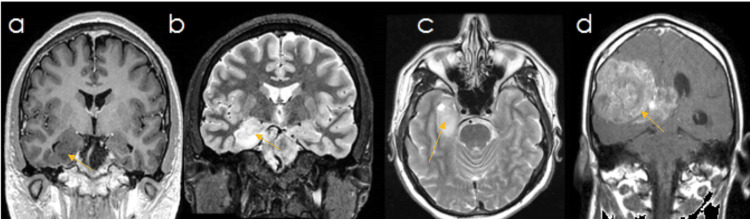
Radiological progression in patient 2 MRI: magnetic resonance imaging; FLAIR: fluid-attenuated inversion recovery Radiological progression in a two-month follow-up in case 2: (A) T1-weighted coronal MRI with hypointense lesion pointed in yellow. (B) T2-weighted coronal MRI with a hyperintense lesion pointed in yellow. (C) T2-weighted axial MRI with a hyperintense lesion pointed in yellow. (D) T2-weighted FLAIR axial MRI with a hyperintense diffuse lesion pointed in yellow
